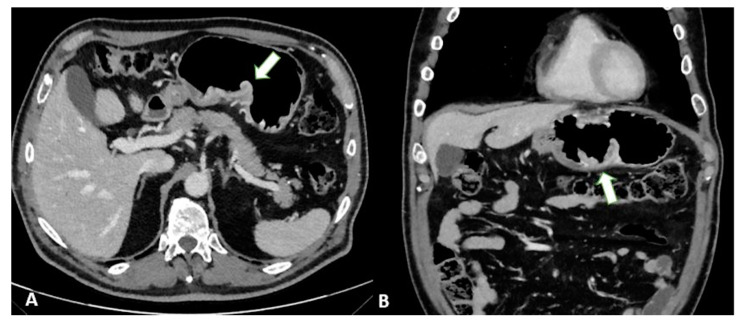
T3 gastric cancer in a 68-year-old female patient. Contrast enhanced CT with gastric distension using air. (A) Axial 2D image and (B) Coronal 2D image in the portal venous phase with distension of the gastric lumen with air showing a semicircumferential tumor (white arrows) of the low body of the stomach with ulcerations.
